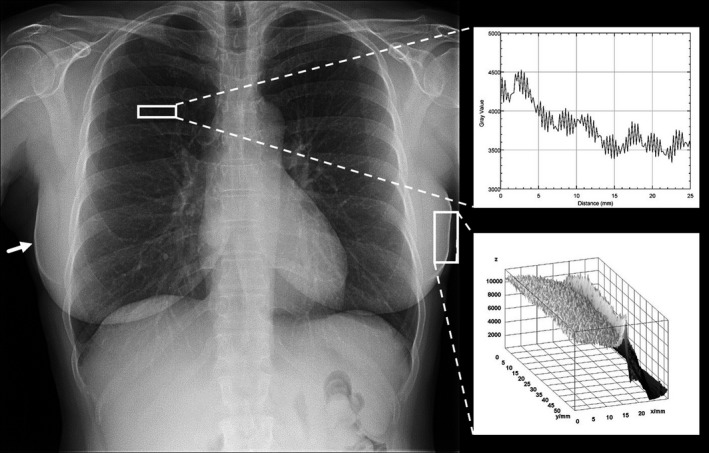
PA chest radiograph that prompted radiologist's image quality complaint on June 1, 2015, for prominent grid lines. Rebound artifacts — also known as “Uberschwinger” artifacts^16^, ^17^, ^18^ — were noted as indicated by the arrow. This is also seen in exaggerated contrast of some cortical bone. Upper right inset: line profile shows exaggerated grid lines in image. The prominent beat frequency corresponds to the aliased frequency of the grid. Lower right inset: surface plot of one of the rebound artifacts.
